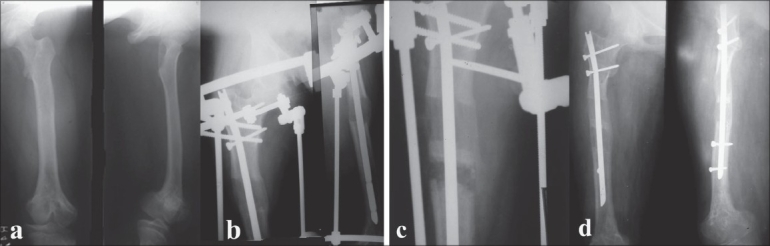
25 year old lady had 12 cm shortening of her femur due to growth arrest. (a) AP and lateral X-rays of thigh including hip and knee. (b) LON method with rapid bone formation lead to premature consolidation at 3 cm. (c,d) A repeat corticotomy was done with the nail in situ which allowed lengthening till 6 cm when a repeat premature consolidation did not allow equalization of limb lengths. Part of the problem was also with the low initial length of the nail which could not have permitted more length
